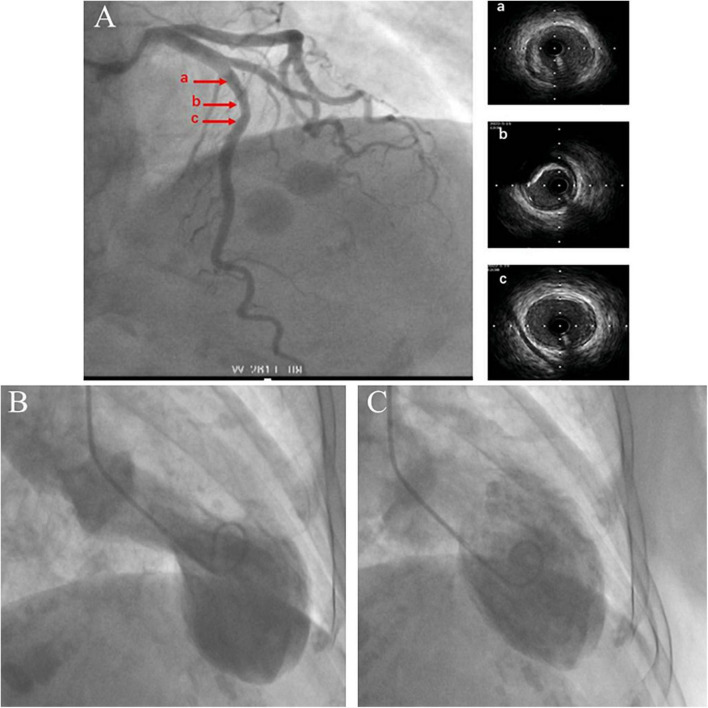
Coronary angiography and intravascular ultrasound show 30% stenosis of the left anterior descending coronary artery with no plaque rupture or dissecting hematoma. The a, b, and c arrows on the left correspond to the ultrasound positions labeled a–c on the right (A). Left ventricular angiography shows a takotsubo contractile pattern with apical dyskinesis and basal hyperkinesis (B,C). (B) Systole. (C) Diastole.
